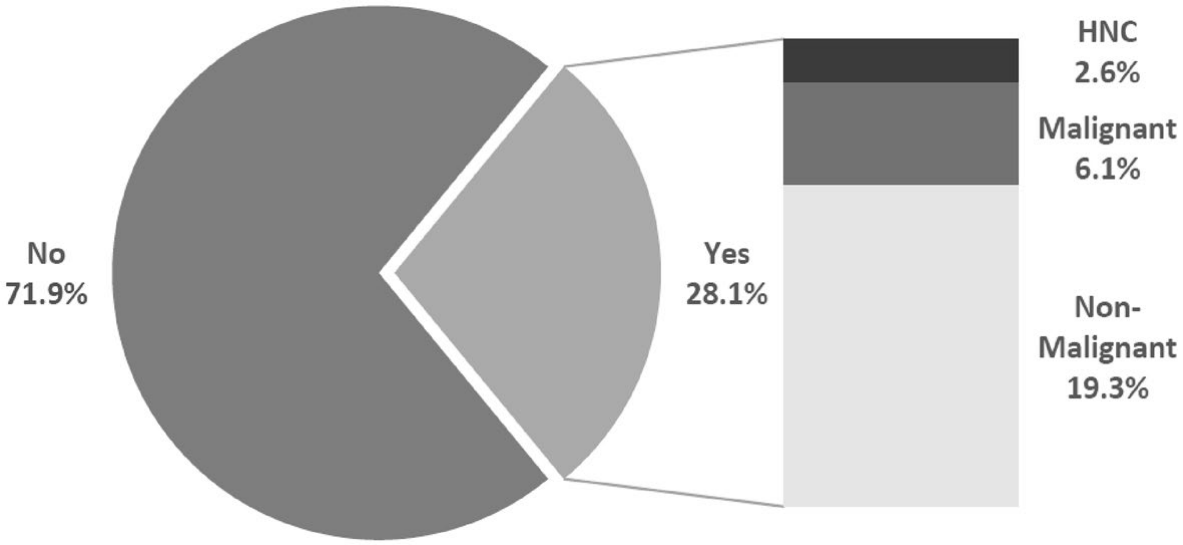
Additional Findings in PET/CT. In 82 (71.9%) patients there was no suspicion described in PET/CT besides HNC. In 29 (25.4%) cases of new diagnosed HNC PET/CT showed an additional finding that led to further investigation. In 7 (6.1%) of these cases the additional finding turned out to be a further malignancy. In 3 (2.6%) further cases PET/CT was performed as secondary staging of another malignant disease and HNC was the additional finding.
